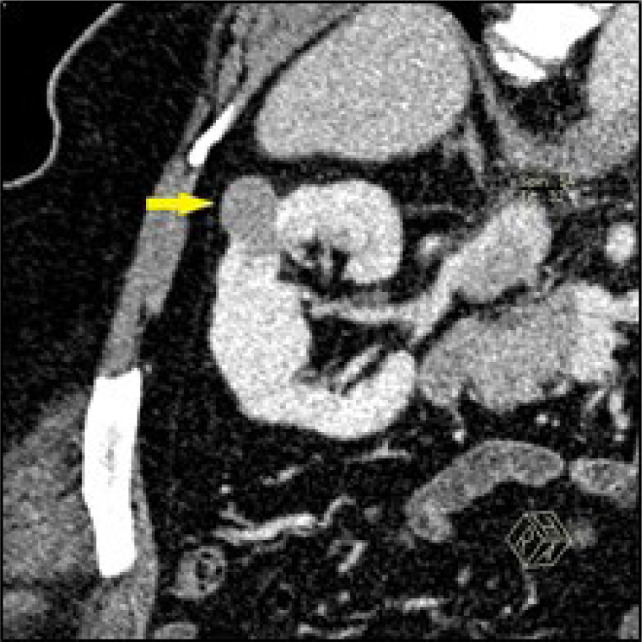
Coronal oblique reformatted CT image of the right kidney in nephrographic phase in a 36-year-old female, showing a 31 mm solid exophytic mass lesion (Arrow) with evident angular interface sign. The mass was enucleated and confirmed lipid-poor angiomyolipoma.
